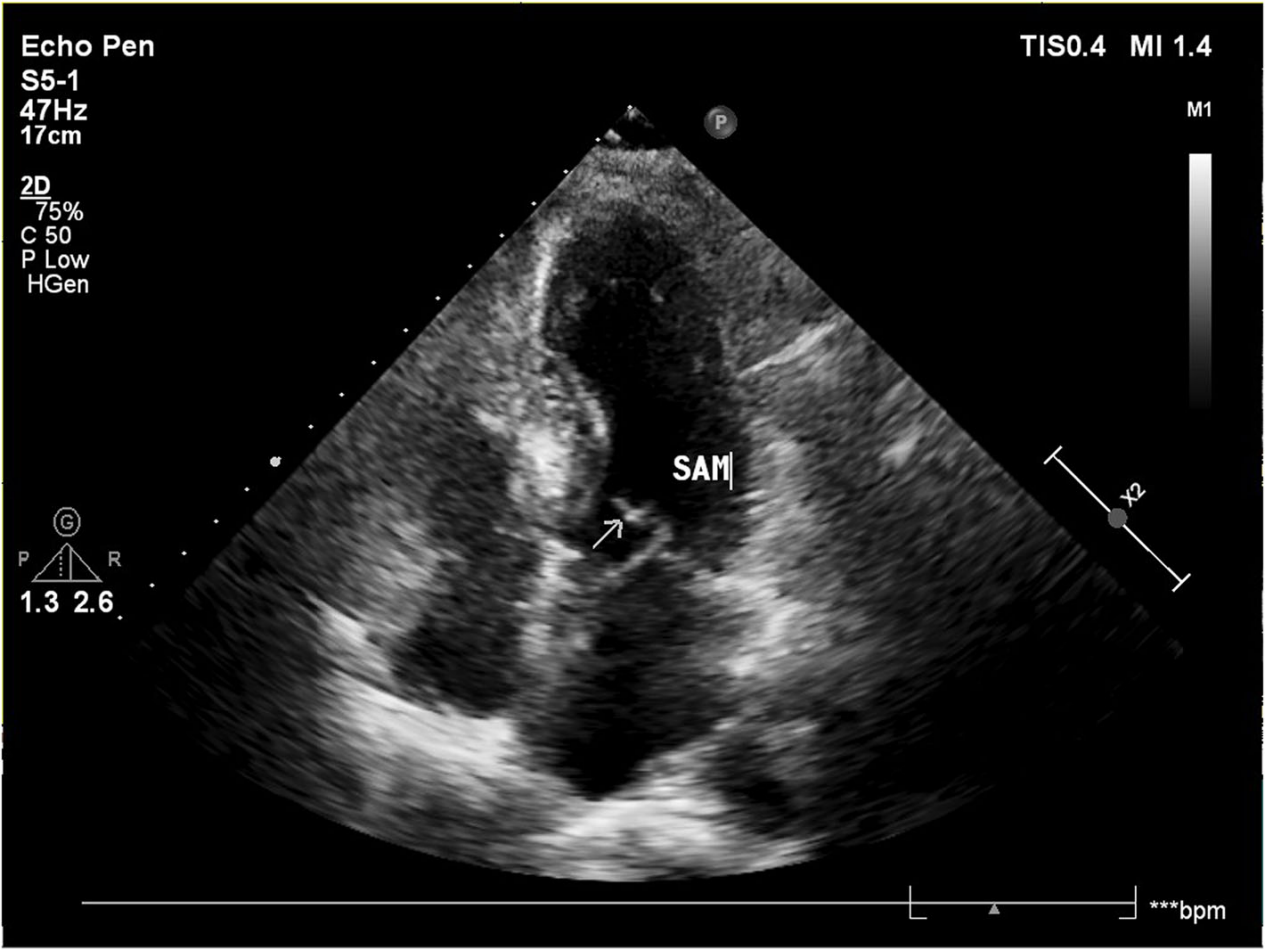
Septal hypertrophy with SAM showed in ultrasonography and no sign of pericardial effusion
